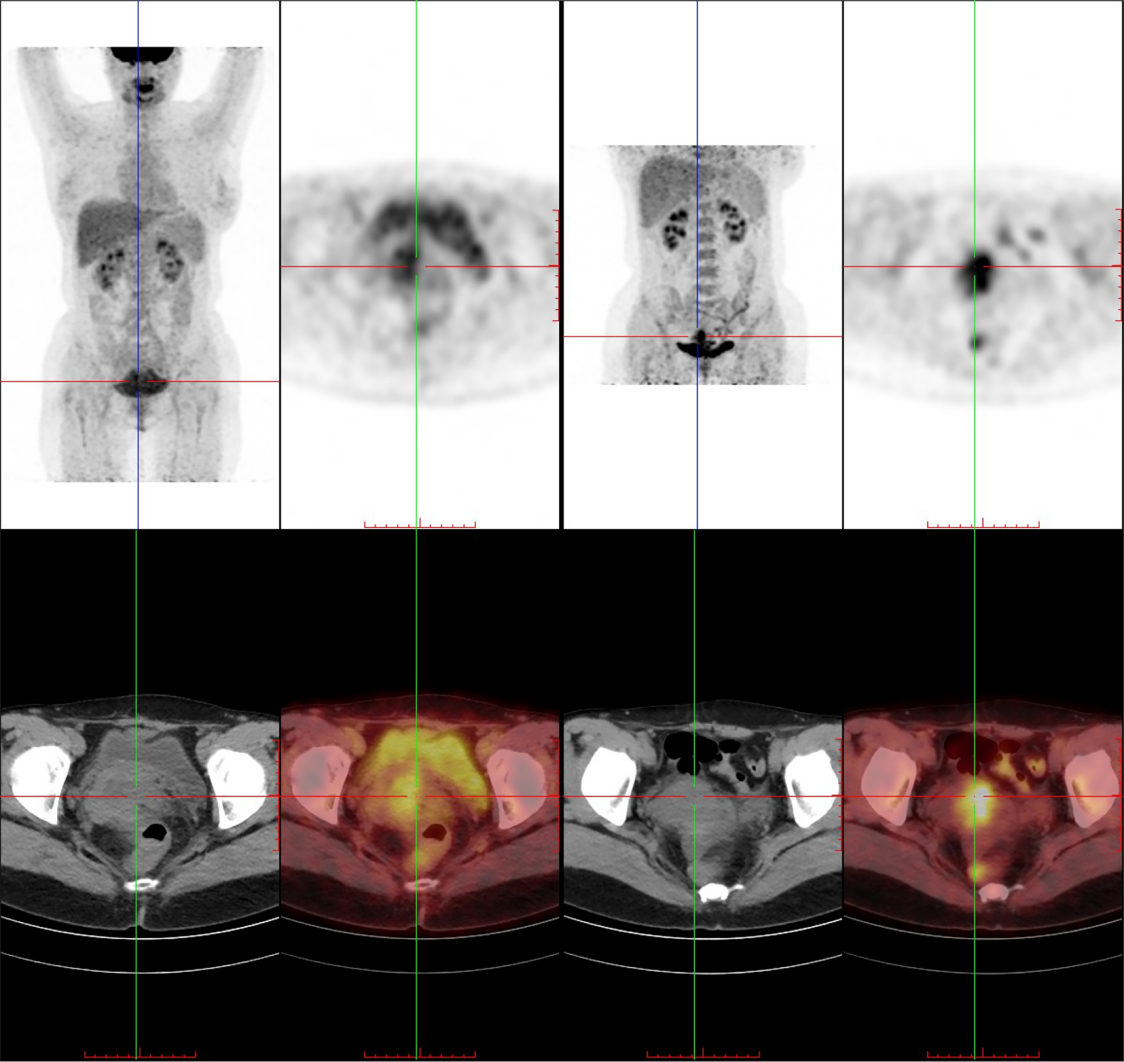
The cervix is widened with mass radioactive concentration foci, with a range of about 38 mm * 24 mm, SUVmax 4.0, delay 5.2. Radioactive concentration can be seen in the lower part of the uterine body, SUVmax 3.9, delay 5.6. Uterine effusion.
